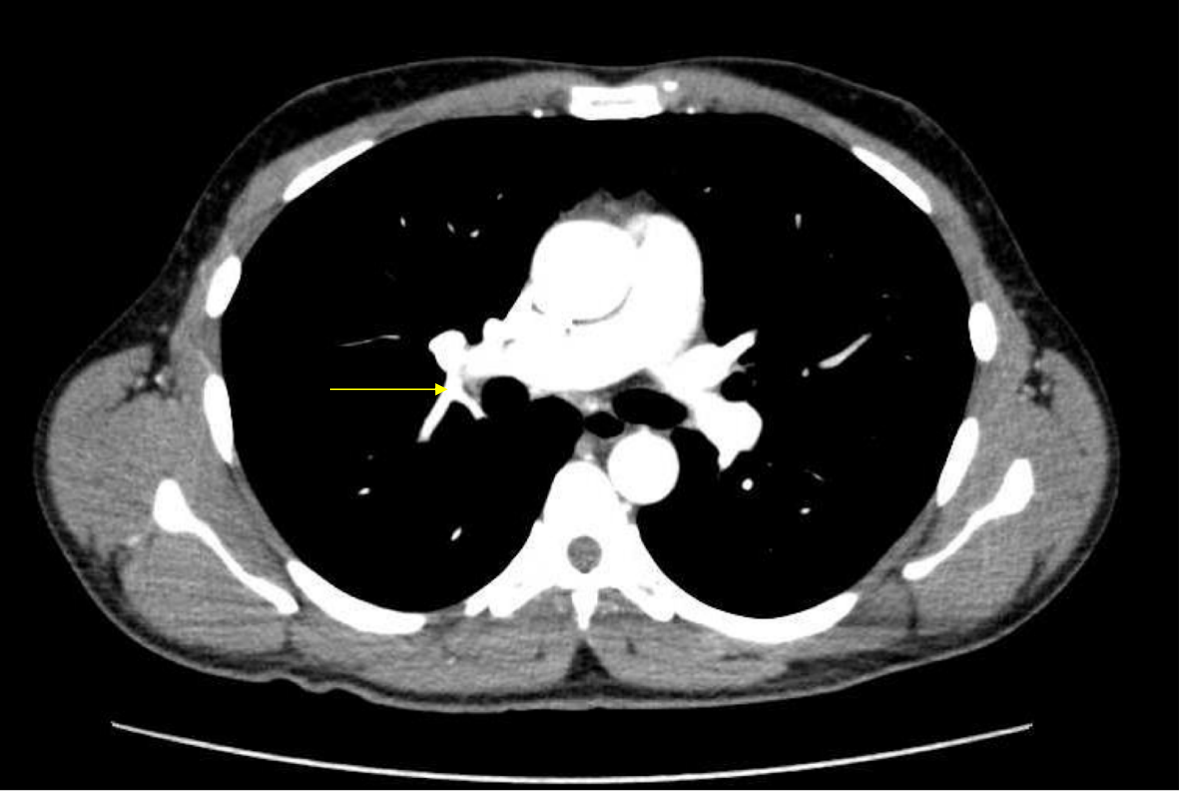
Contrast-enhanced computed tomography 7 days after the initiation of anticoagulation therapy. The *yellow arrow* indicates reduced thrombosis
